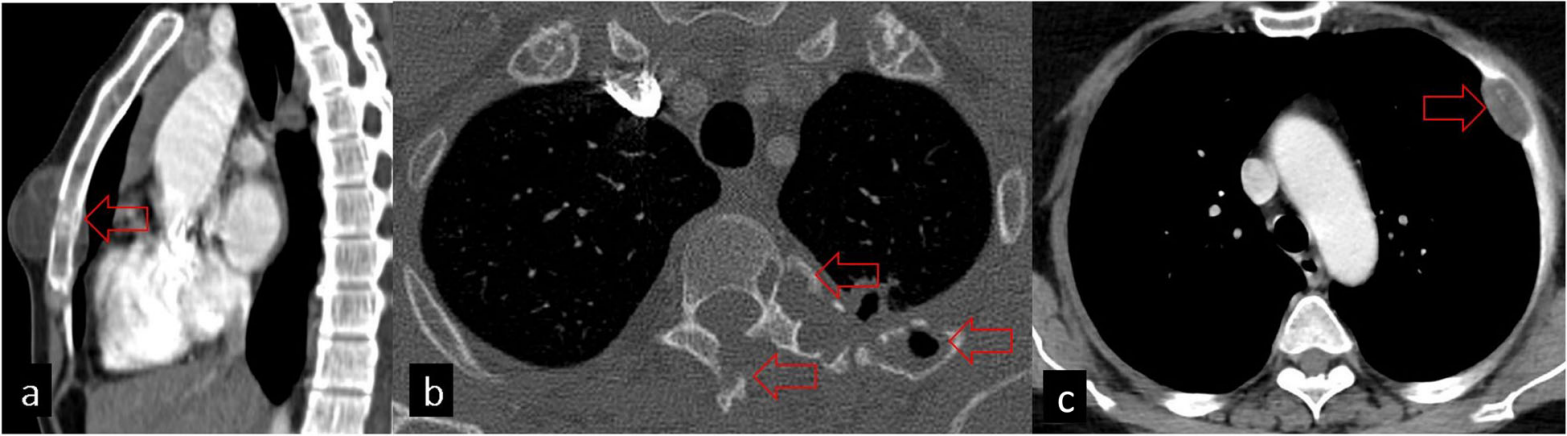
Bone hydatid cysts and differential diagnosis. a Axial chest CT image shows an HC located anteriorly adjacent to the sternum causing sternal cortical erosion (red arrow) b Axial CT image in the bone window shows the destruction of the vertebra and adjacent costa (red arrows) secondary to HC. c Costa destruction secondary to lung cancer is seen (red arrow)
